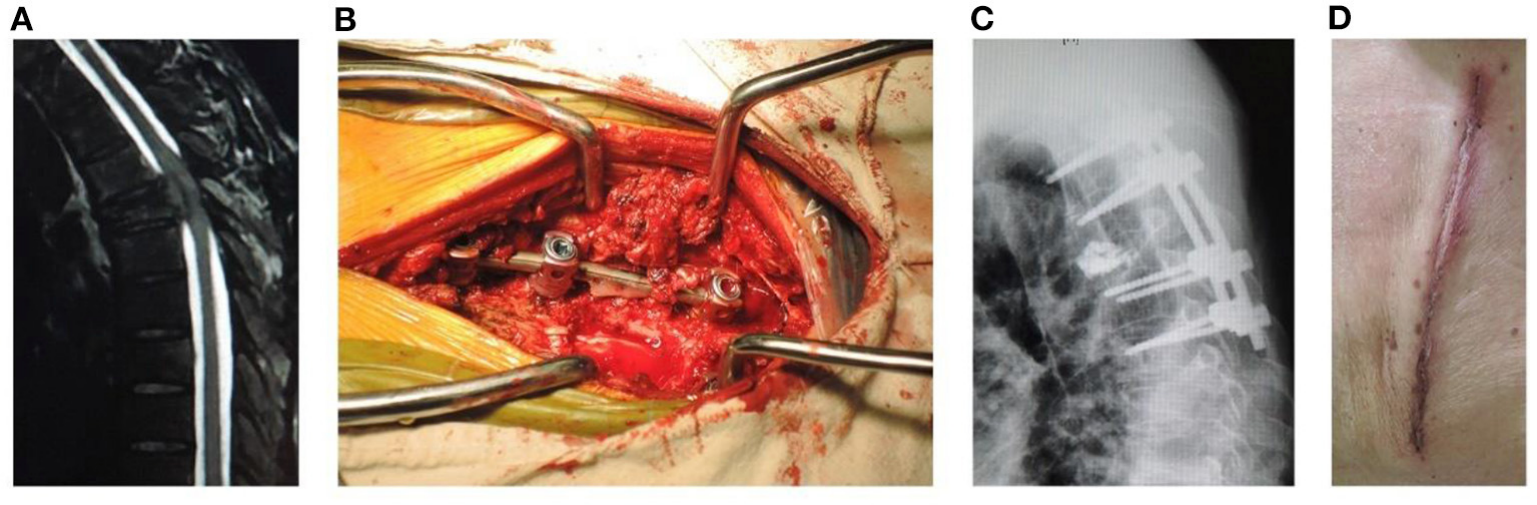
A male patient of 53 years old with T5 metastasis and neurologic compression from HCC had back pain VAS 8, ASIA C, Tomita 7, modified Tokuhashi 12, ESCC grade 3, and SINS 14. He underwent open surgery for internal fixation, PVP, neurologic decompression, and partial tumor resection, with an operation duration of 180 min, intraoperative blood loss of 800 ml, incision length of 14 cm, and stay in hospital of 11 days. (A) Sagittal MRI showed metastasis of T5 with neurologic compression. (B) Intraoperative photography. (C) Sagittal X-ray showed that the position of pedicle screws was good and no leakage of cement into the spinal canal occurred. (D) Incision after open surgery.
